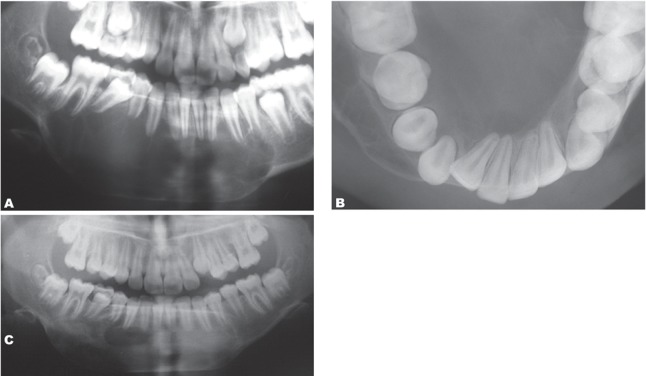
A 10-year-old girl presenting with painful swelling in the lower jaw.( A. Panoramic view shows a multilocular lesion in the symphysis region of the mandible. B. Occlusal projection shows considerable buccal and lingual expansion. Note the septa which are straight and at right angles to the periphery, mimicking central giant cell granuloma appearance. C. Follow-up panoramic X-ray taken one year after the surgery displays resolution of the lesion.)
